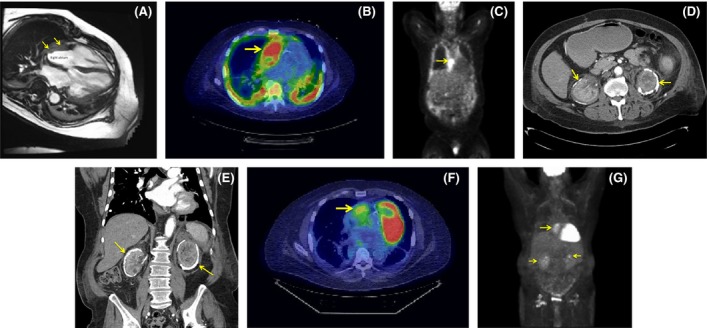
(A) Magnetic resonance imaging of the chest, T2 weighted, fast field echo image, horizontal long axis view showing an atrial mass infiltrating the right atrial wall (arrows). (B) PET‐CT, axial view and (C) coronal view, showing FDG increase uptake in the right atrial mass. (D) CT scan of the abdomen with contrast, axial view and (E) coronal view, showing severe perinephric dystrophic calcification (arrows). (F) Follow‐up PET‐CT, axial view, and (G) coronal view, reveal moderate decrease in the soft tissue density and FDG uptake in the right atrial infiltrative mass. Perinephric thickening and dystrophic calcification unchanged (arrows).
